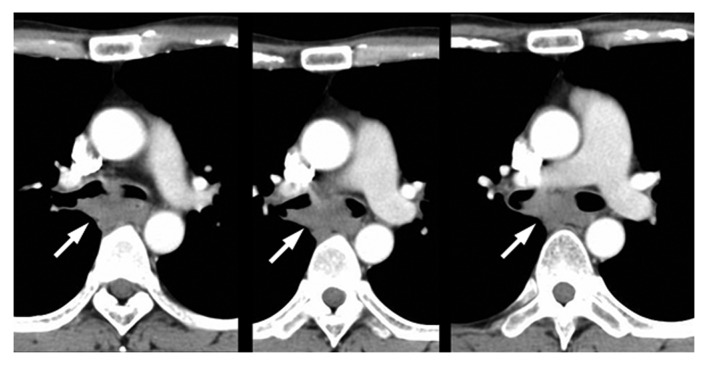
Contrast-enhanced computed tomography (CT) scan on admission showing subcarinal lymphadenopathy and an endobronchial tumor at the main carina.
